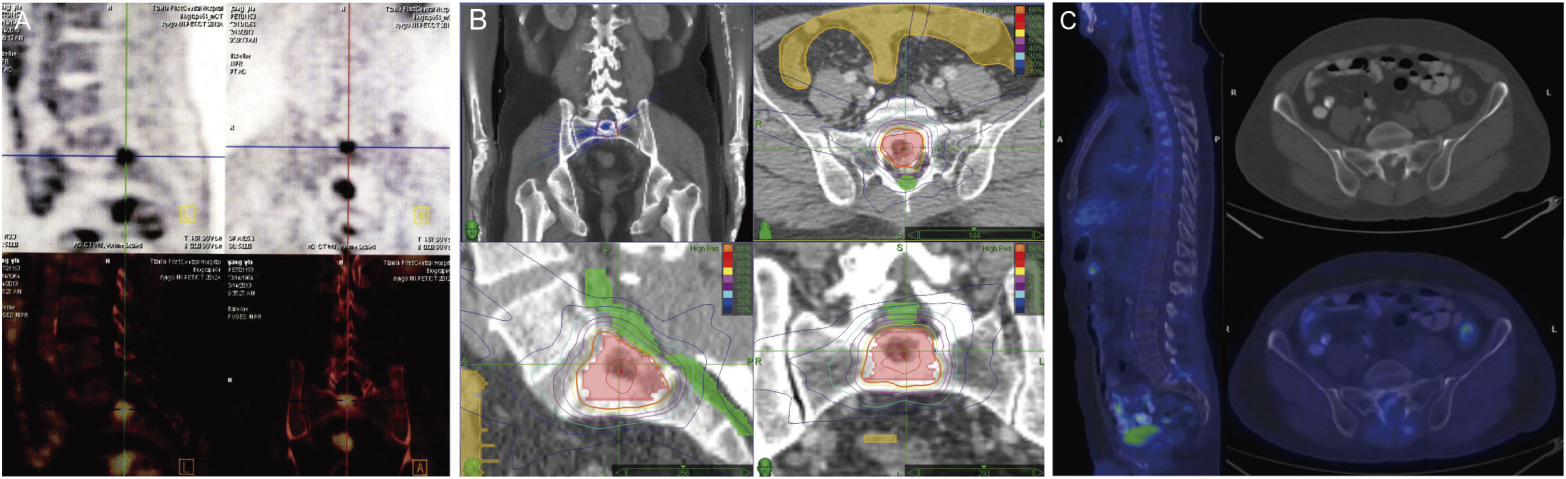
Examples of dose distributions and treatment outcome for one patient. (A) PET-CT scan before SRT. (B) Treatment plan with 30Gy in three fractions. (C) PET-CT scan 18 months after SRT.
